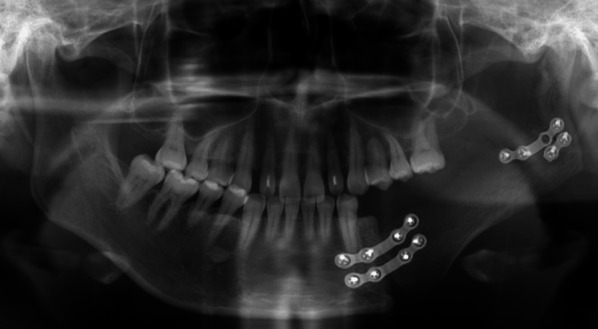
Panoramic radiograph after segmental mandibulectomy and reconstruction
